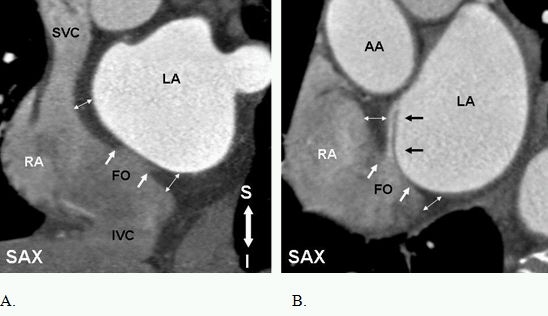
Anatomy of the interatrial septum and patent foramen ovale (PFO). Short axis (SAX) images perpendicular to the interatrial septum show the fossa ovale (FO) in (A) and a well structured PFO in (B). The septum primum (black arrows in B) is fused to the inferior rim of fossa ovale (FO) and extends superiorly as a flap. The superior and the inferior rims (white arrows) of the FO are formed by infolding of the right atrial wall [interatrial groove (IAG) or the septum secundum]. IAG (double headed arrows) contains extracardiac fat. The infolding of the right atrial wall overlaps the flap of septum primum, forming a narrow tunnel through which a probe can be passed (51). AA= ascending aorta, LA=left atrium, RA=right atrium, S=superior, I=inferior. Reproduced  from: Saremi F, Tafti M. The role of computed tomography and magnetic resonance imaging in ablation procedures for treatment of atrial fibrillation. Semin Ultrasound CT MR. 2009;30:125-56, Copyright (2009), with  permission from Elsevier.
